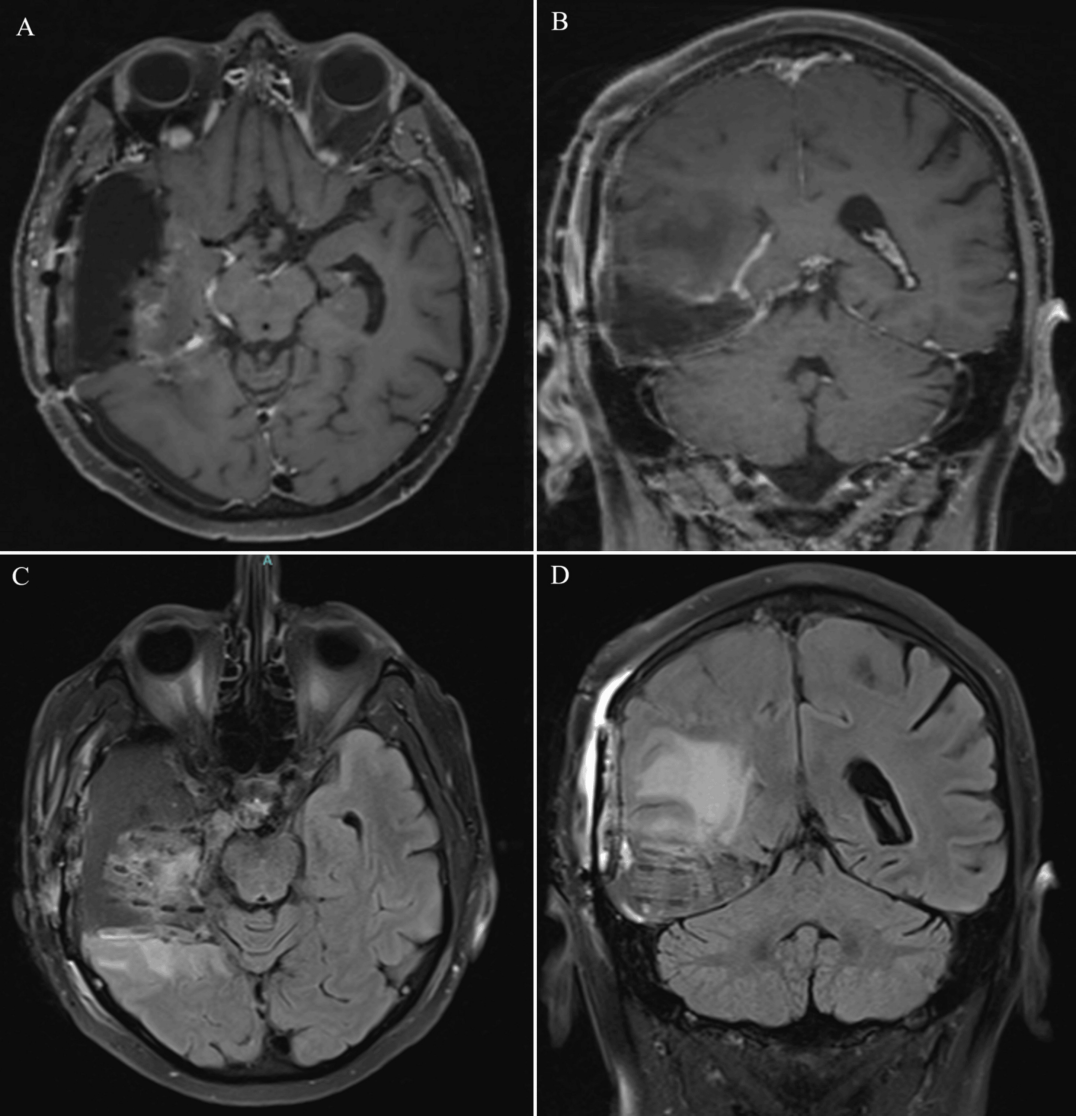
Postsurgical imaging after the patient’s second resection and first GT implant placement for tumor recurrence: (A) Axial and (B) coronal T1-weighted MRI with contrast, along with (C) axial and (D) coronal FLAIR sequences, demonstrating postoperative changes and implant placement GT, GammaTile
